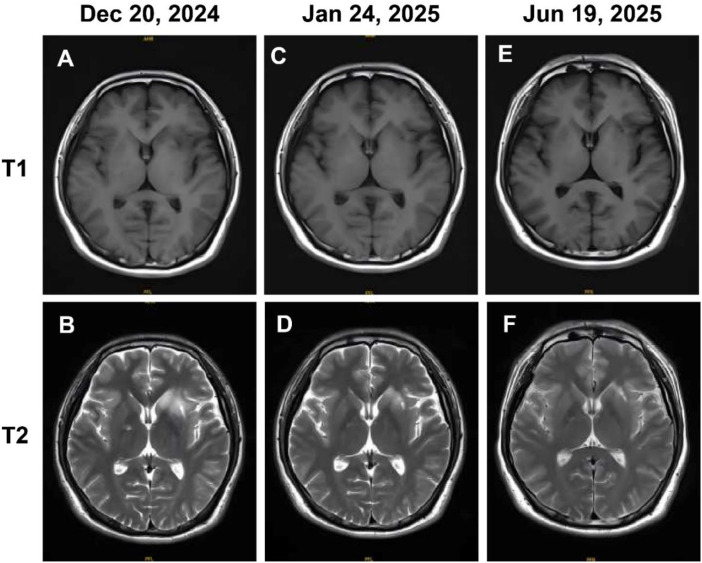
Brain MRI images of patient 1. (A, B) Images obtained prior to intravenous methylprednisolone and tocilizumab therapy. (C, D) Follow-up MRI scans conducted a month post-treatment. (E, F) Follow-up images acquired six months post-treatment with regular tocilizumab infusions.
